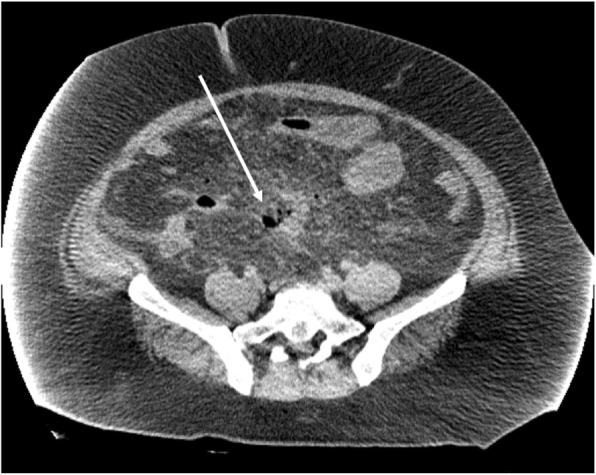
Abdominal computed tomography showing free air around the sigmoid colon
